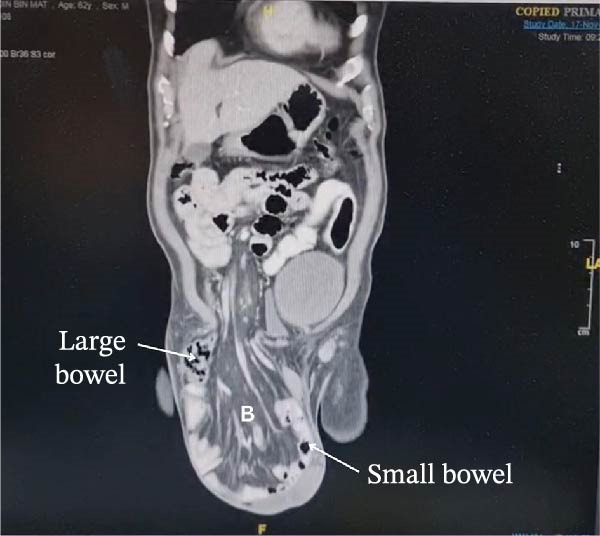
Coronal plane CT scan showing small bowel and large bowel contained in the inguinal hernia (B).
